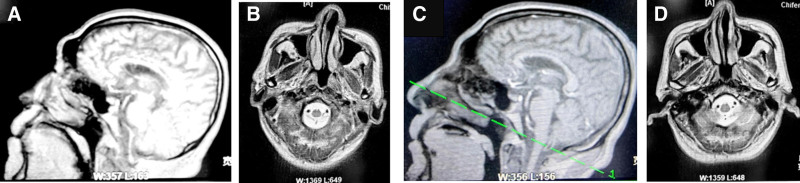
The result of MRI. (A, B) The MRI results of September 21: an abnormal signal is noted in the occipital horn of the right lateral ventricle, along with meningeal enhancement. Based on the medical history, the findings are suggestive of purulent meningitis accompanied by a small amount of fluid accumulation in the ventricles. (C, D) The MRI results of October 6: meningeal enhancement is noted in the right frontoparietal region. Based on the medical history, the findings are consistent with purulent meningitis. The conclusion is largely consistent with the findings from September 21.
